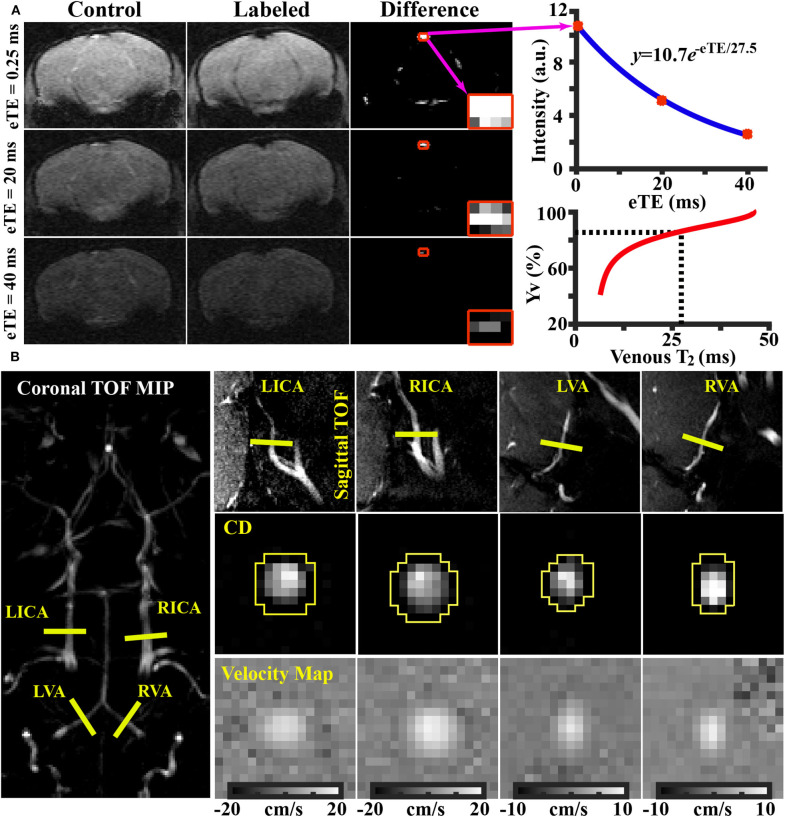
Representative results of (A) TRUST and (B) PC MRI. (A) Left panel: control, labeled, and difference images from TRUST MRI obtained at different effective TE (eTE) values. The sinus confluence is shown in red squares. Right upper panel: Blood signal in the sinus confluence as a function of eTE. Blue curve indicates fitting results. Right lower panel: Y_v_-T_2_ calibration plot used to convert blood T_2_ to oxygenation (33). (B) Left panel: imaging slice locations of PC MRI overlaid on a TOF image. Right upper panel: PC MRI imaging locations overlaid on respective sagittal TOF image. Right middle panel: complex difference (CD) images of the four arteries. Right lower panel: velocity maps of the four arteries.
